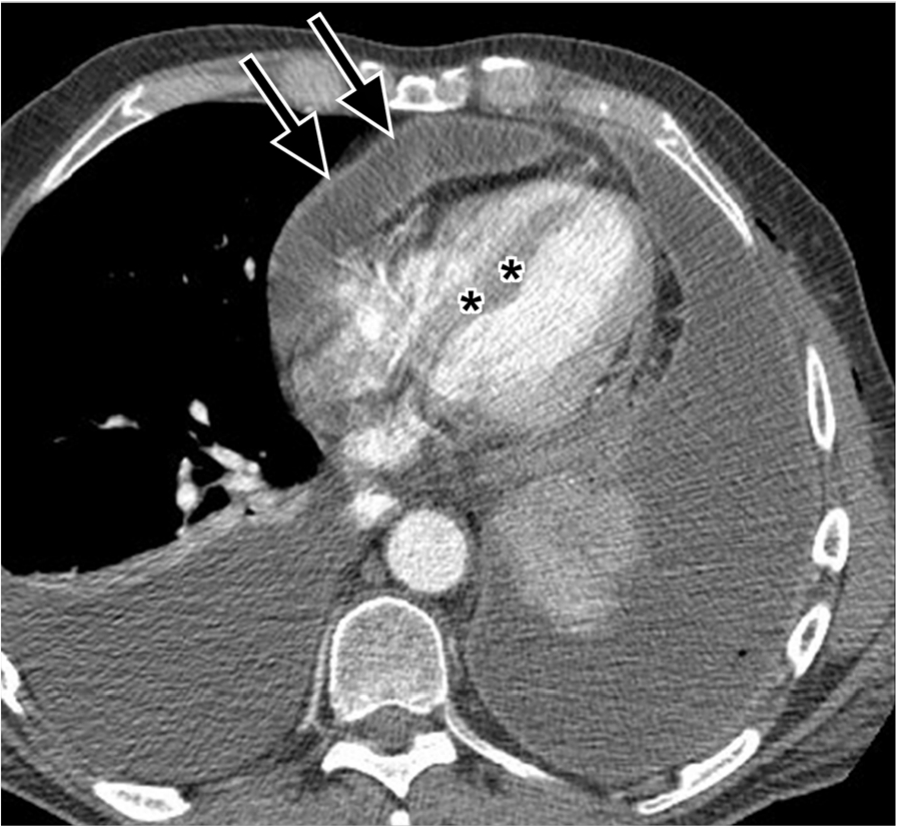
Axial contrast-enhanced CT shows thickening of the visceral and parietal pericardium (black arrows) with fluid in between the pericardial layers in a 77-year-old male patient being investigated for loculated pleural effusion. This pericardial thickening and fluid are resulting in constrictive physiology (constrictive pericarditis), evidenced by a decreased volume, cone-shaped right ventricle, and a sigmoid-shaped interventricular septum (black asterisks)
